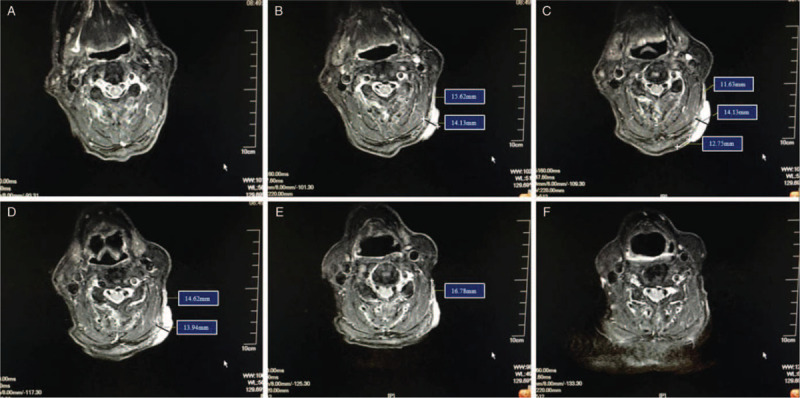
Magnetic resonance imaging of aggressive CD30+ primary cutaneous anaplastic large cell lymphoma. The T2 sequence revealed a lesion that protruded (white arrow) from the skin. The thin areas (yellow arrow) represented the subcutaneous part of the tumor. The images in A–F revealed the extent of invasion by depth and circumference.
